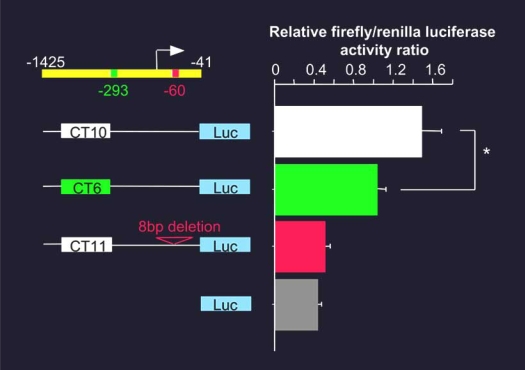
Transcriptional activities of the indicated (CT)n and 8-bp deletion reporter constructs in human aortic smooth muscle cells. Shown are the mean firefly (Luc)/renilla luciferase activity ratios. Note that the GC-A promoter containing (CT)n=6 or the 8bp deletion drove significantly less transcriptional activity than the promoter containing (CT)n=10. Modified from reference [34].
